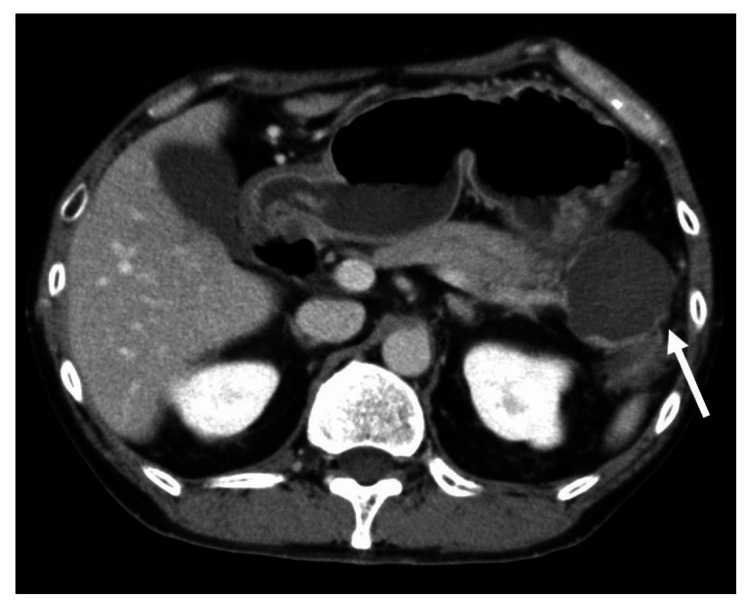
Initial computed tomography. Computed tomography revealed a cyst measuring 5 cm in diameter at the tail of the pancreas (arrow). No bile duct stones were found.
